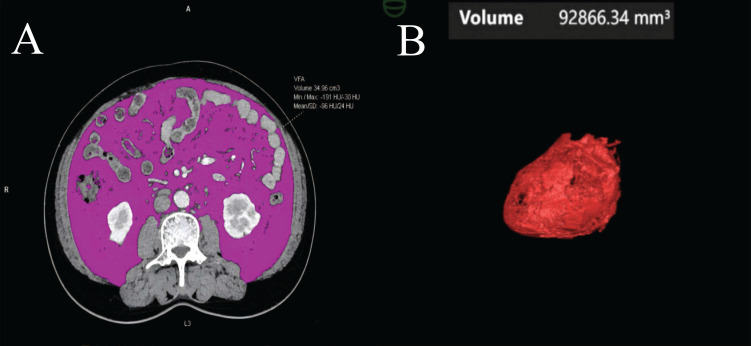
Visceral fat and epicardial adipose tissue volume measurements from a CT scan. (A) The visceral fat area (VFA) was measured at the third lumbar vertebra. Within this region of interest, pixels between -190 and -30 Hounsfield Units were accounted as fat. (B) After 3-dimensional reconstruction, EAT volume was calculated by summation of all pixels accounted as fat.
